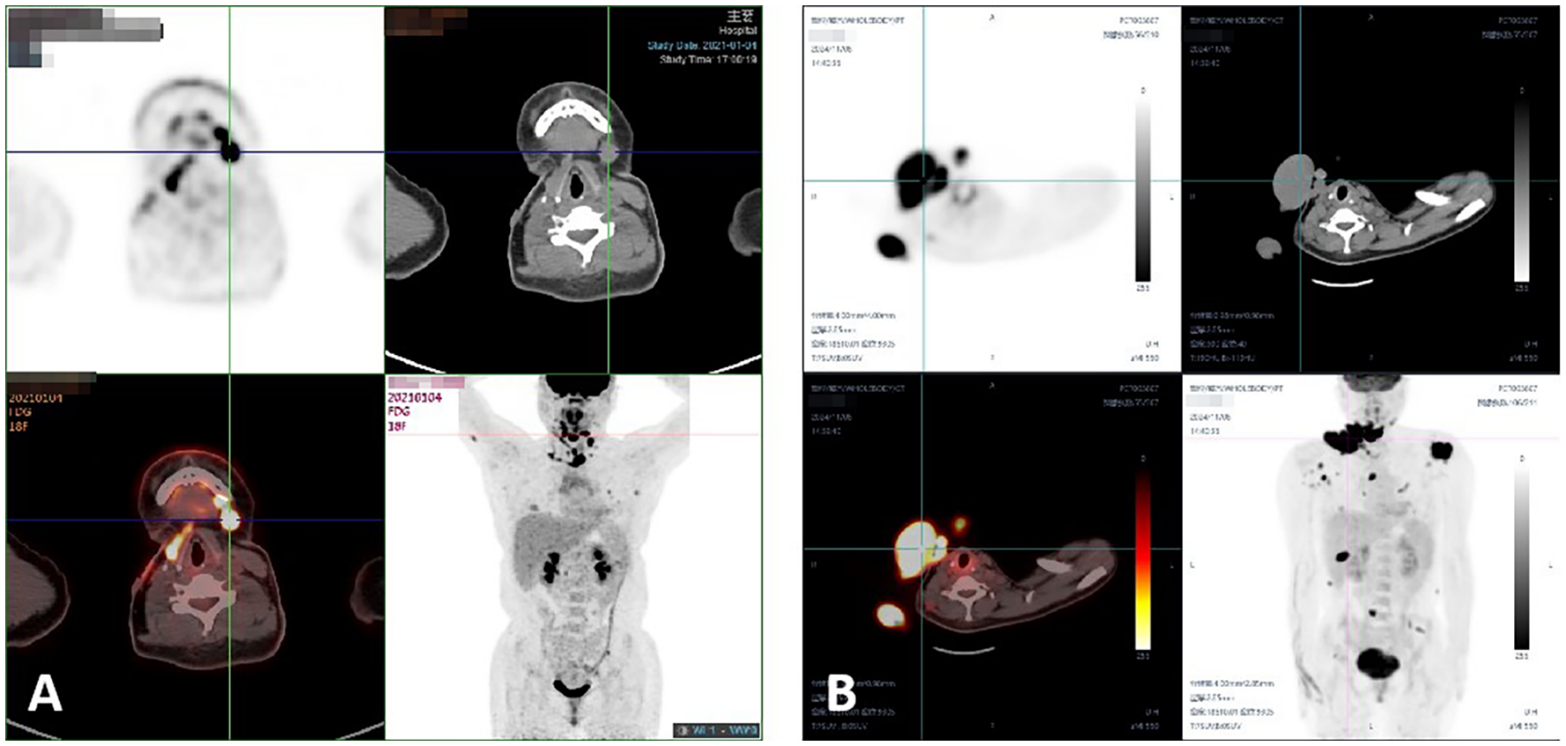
(A) The patient’s PET-CT results indicated multiple subcutaneous metastases in January 2021. (B) The patient’s PET-CT results revealed multiple subcutaneous and lung metastases in November 2024.
